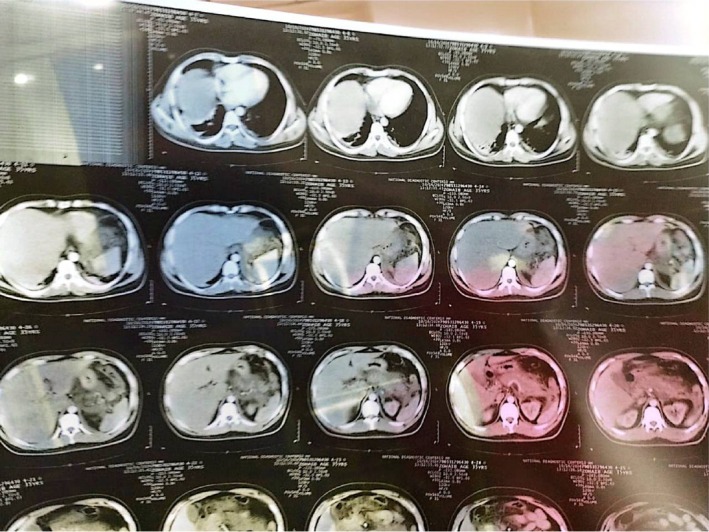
Upper Abdominal CT Sections Showing hepatomegaly (enlarged liver) and gallbladder wall thickening in the absence of gallstones (cholelithiasis).
